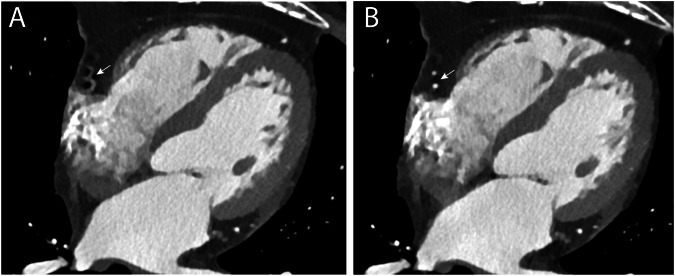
Standard reconstruction vs. motion compensation reconstruction of the coronary CTA image. Clinical examples of a standard coronary computed tomography angiography image (physiologic cardiac phase 78% RR interval) that (A) contains severe motion artifacts in the right coronary artery (non-diagnostic segment, white arrowhead) with standard reconstruction (SR) and (B) with motion compensated reconstruction (MCR) that reduced the motion artifact, enabling accurate assessment of this segment (Likert score 3)
